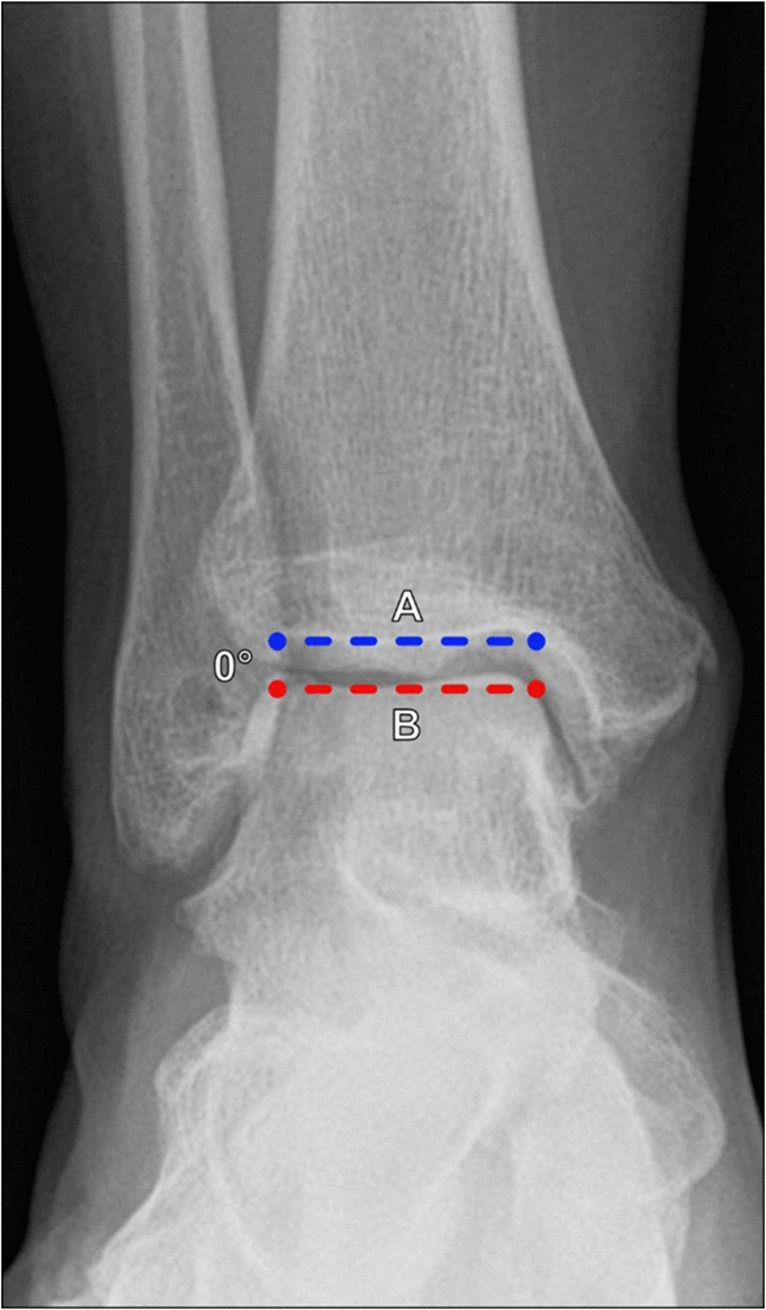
Measurement of the talar tilt angle on an AP radiograph of the ankle. The angle is formed from a line tangent to the tibial plafond (A, blue line) and a line along the talar dome (B, red line). In practice, accepted normal values in neutral position are < 2° of varus angulation; with supination/inversion stress the angle should measure < 5°. This measurement reflects the integrity of the calcaneofibular and anterior talofibular ligaments
